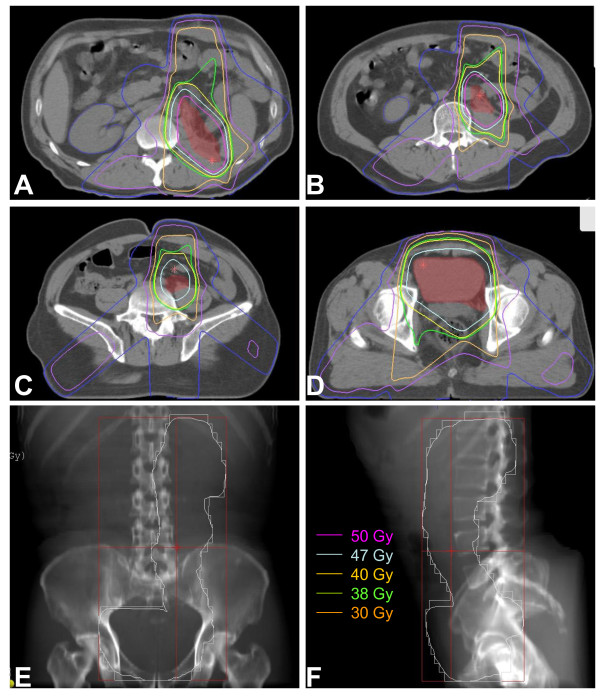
Dose distribution of a patient with renal pelvis cancer and beam arrangements of 0°, 129°, and 229° gantry: (A) renal fossa; (B, C) course of ureter; and (D) bladder. Digitally reconstructed radiograph for views of (E) 0° gantry and (F) 90° gantry. Internal pink and yellow lines represent the CTV50 and CTV40 respectively.
